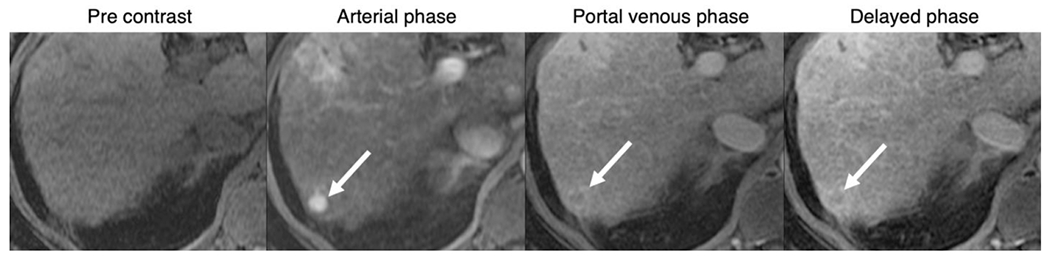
Positive dynamic-AMRI examination: 80-year-old male with HCV cirrhosis, images show a 11 mm observation in segment 7. The lesion has major features of HCC including nonrim APHE, washout and enhancing capsule (arrows) indicating definite HCC (LI-RADS-5). AMRI: abbreviated magnetic resonance imaging; HCV: hepatitis C virus; HCC: hepatocellular carcinoma; LI-RADS: Liver Imaging Reporting and Data System; APHE: arterial phase hyperenhancement
